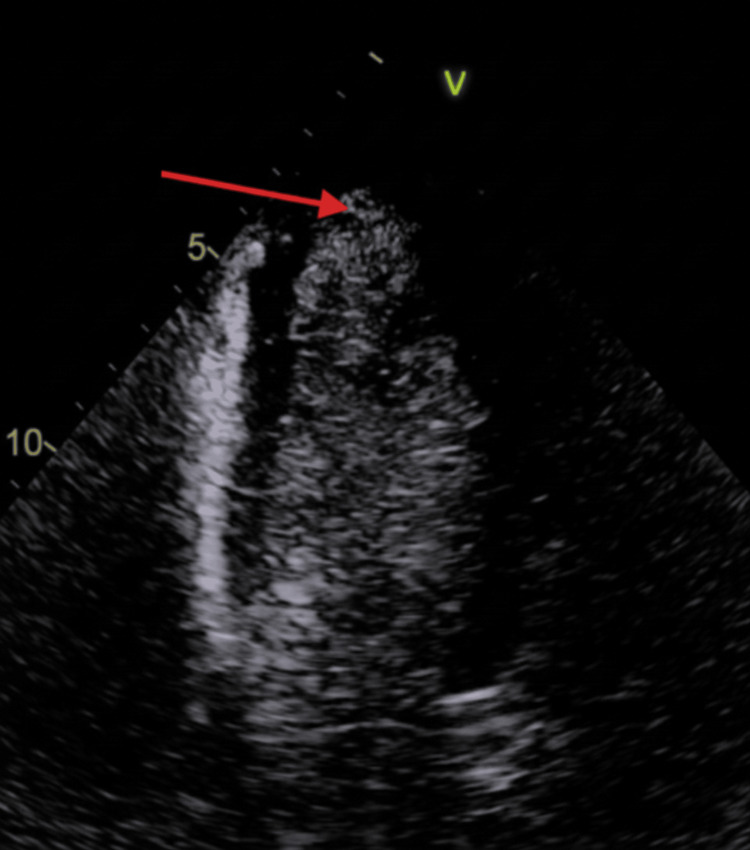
Contrast Transthoracic Echocardiogram Demonstrating Absence of Left Ventricular Thrombus Contrast echocardiogram of the left ventricle with the red arrow indicating the region of interest demonstrating the absence of thrombus.
